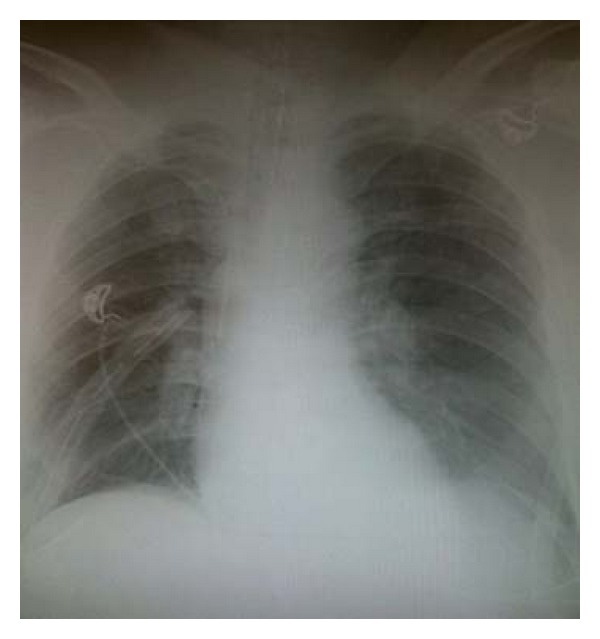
X-ray depicting repair of diaphragm.
